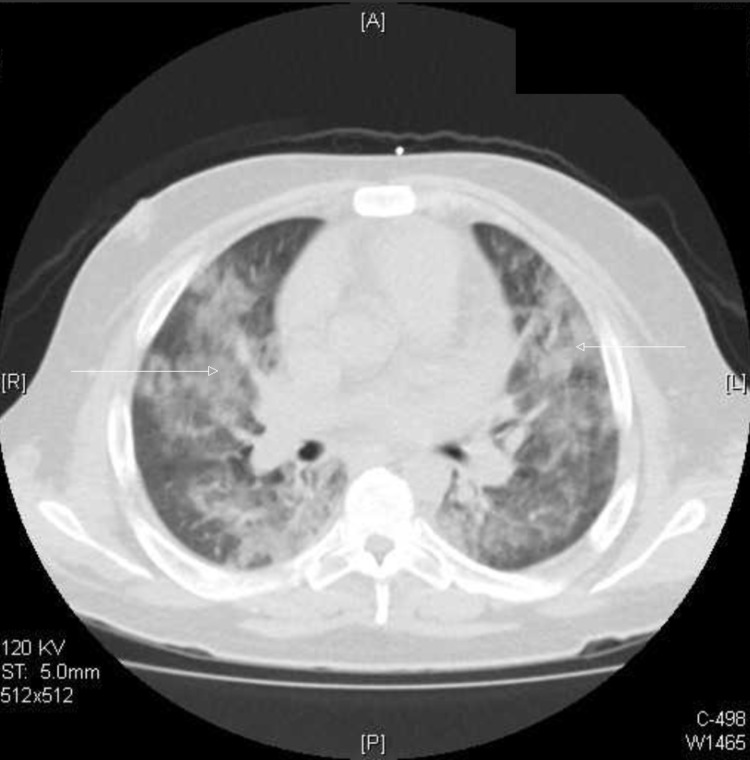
Chest CT scan shows diffuse bilateral patchy opacities (arrows) CT: computed tomography
